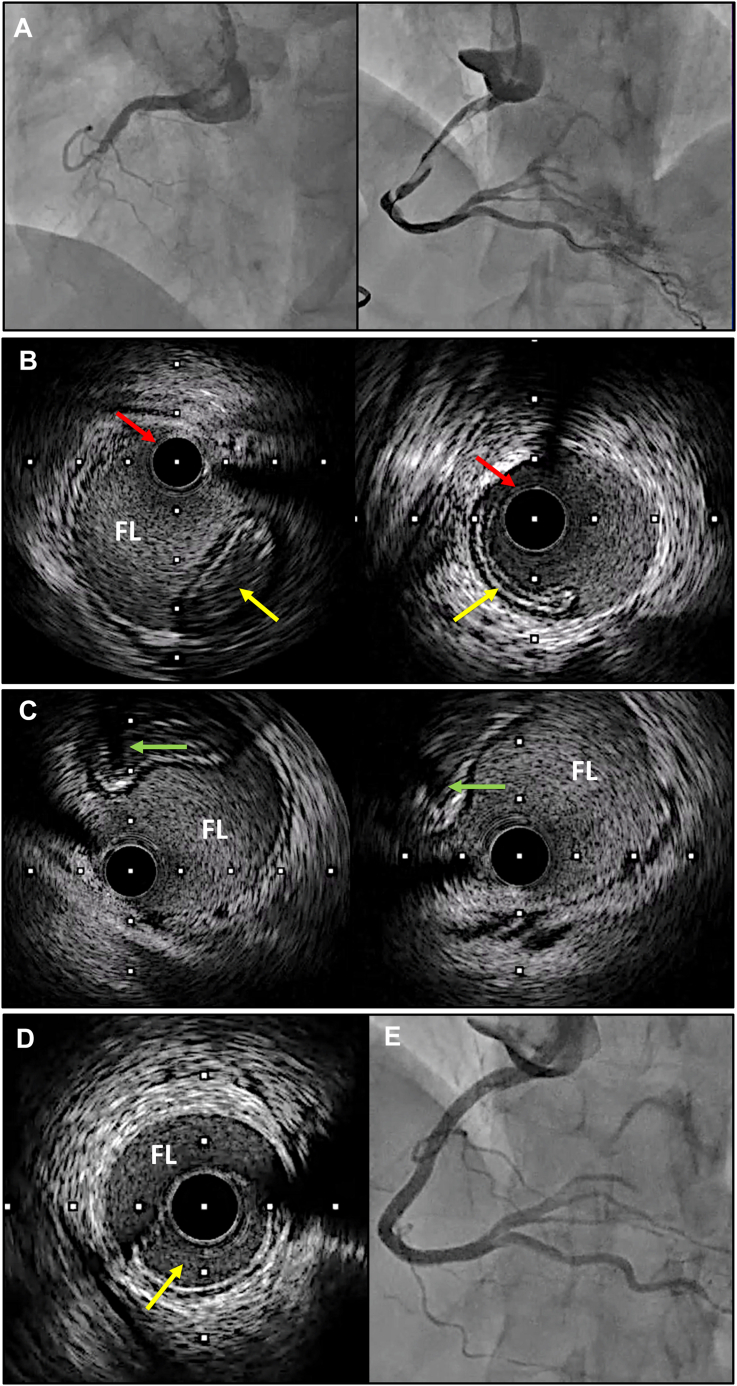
Intravascular ultrasound (IVUS)-guided stenting for iatrogenic aortocoronary dissection. (A) Iatrogenic aortocoronary dissection after guiding-catheter engagement into the right coronary artery. (B) “Apple turnover–like appearance” of the true lumen (TL; yellow arrows) and the first guidewire with the IVUS catheter in the false lumen (FL; red arrows). (C) The second guidewire (green arrows) into the TL at 2 different levels of the right coronary artery, with the posterior shadow cone. (D) The IVUS catheter on the second guidewire insert in the TL (yellow arrow). (E) Final angiogram showing thrombolysis in myocardial infarction (TIMI) 3 coronary blood flow, without extension of the aortic root hematoma.
